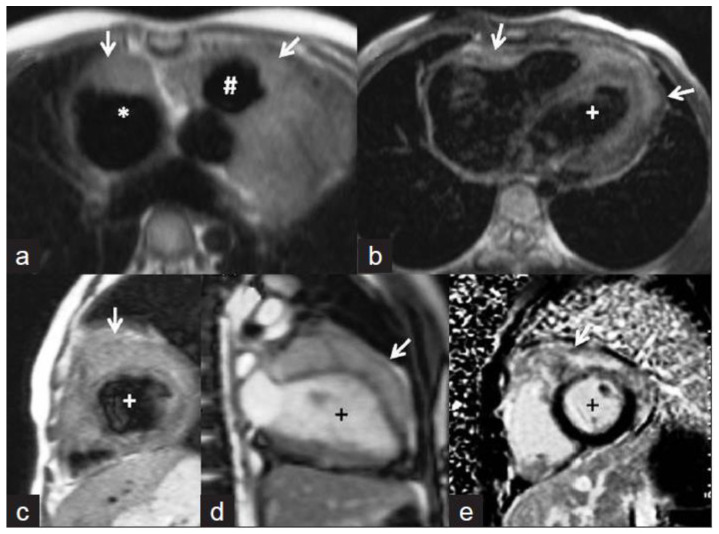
Cardiac magnetic resonance images. (a,b) Axial T1-weighted images showing isointense masses (arrows) along the anterior right atrium (*), right ventricular outflow tract (#), and along both ventricles (+ indicates left ventricle). (c) Short axis T2-weighted image showing that the lesions are mildly hyperintense. (d) Steady-state free precession image revealing the infiltrative nature of the lesion along the left ventricle. The delayed enhanced short-axis image (e) shows heterogeneous enhancement of the mass. The image was adapted from [47].
